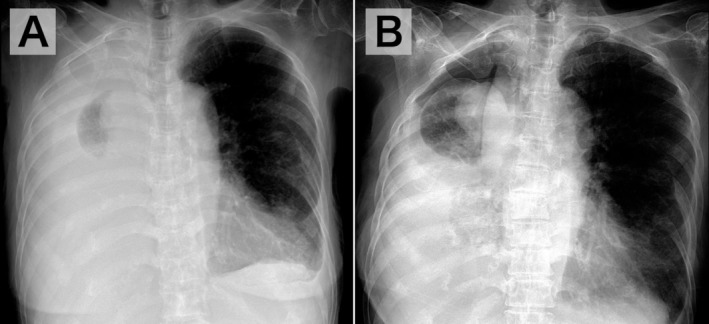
Chest radiographs obtained before (A) and after (B) thoracentesis. (A) Posteroanterior view in the standing position. (B) Anteroposterior view in the seated position. Compared with the pre‐thoracentesis image, the post‐thoracentesis radiograph shows a reduction in right thoracic volume and bronchial deviation toward the procedural side.
